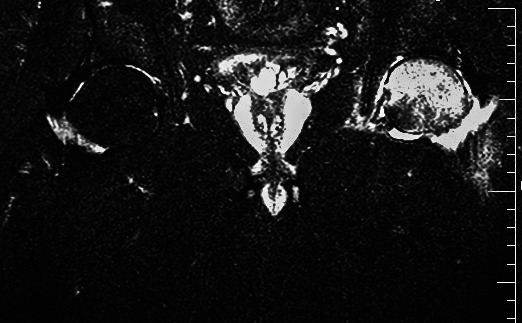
T2‐weighted MRI of the left hip: Coronal image reveals widespread bone marrow edema
